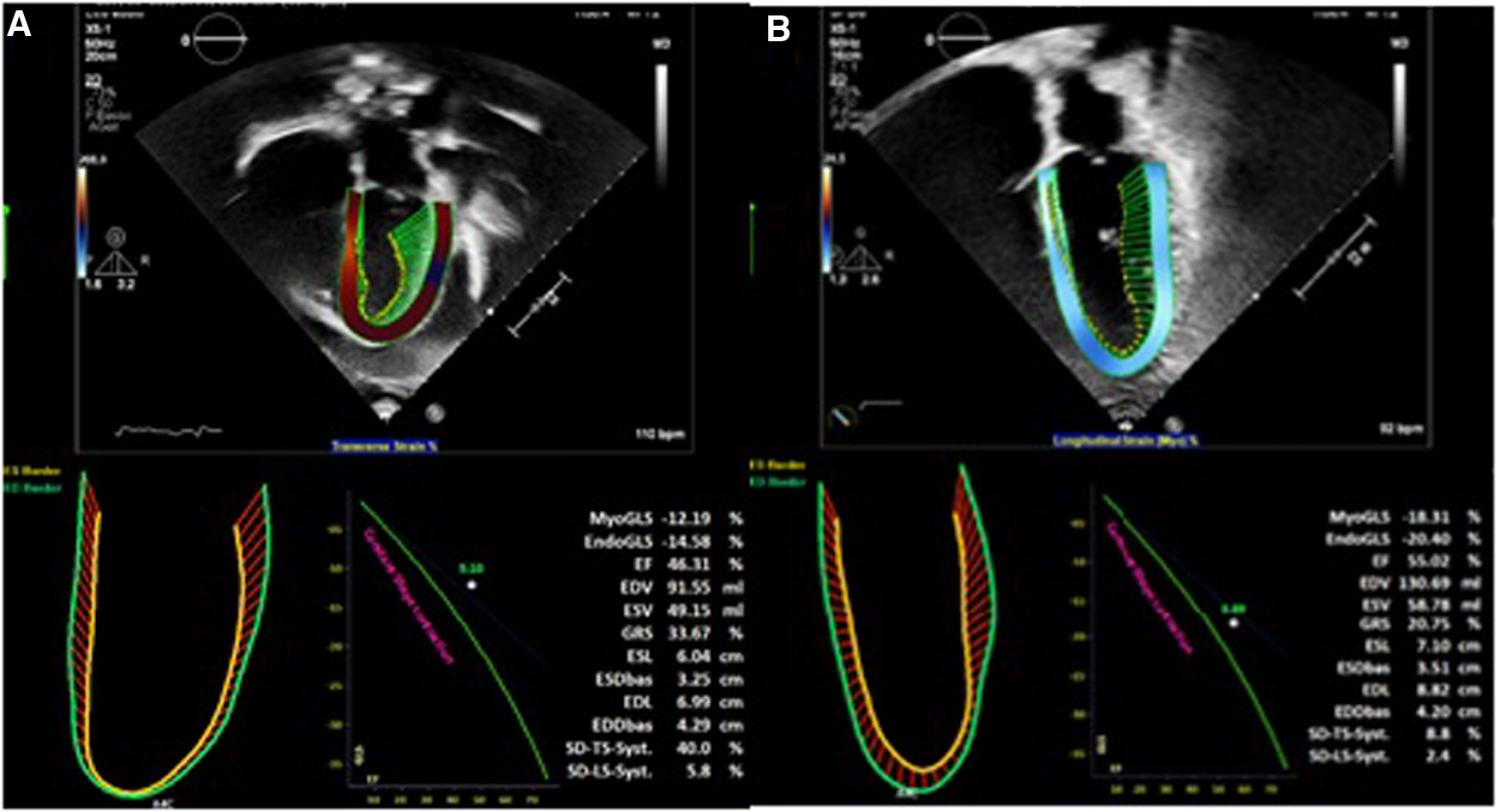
(A) Transthoracic echocardiography before cardiac surgery. (B) Transthoracic echocardiography after cardiac surgery.
